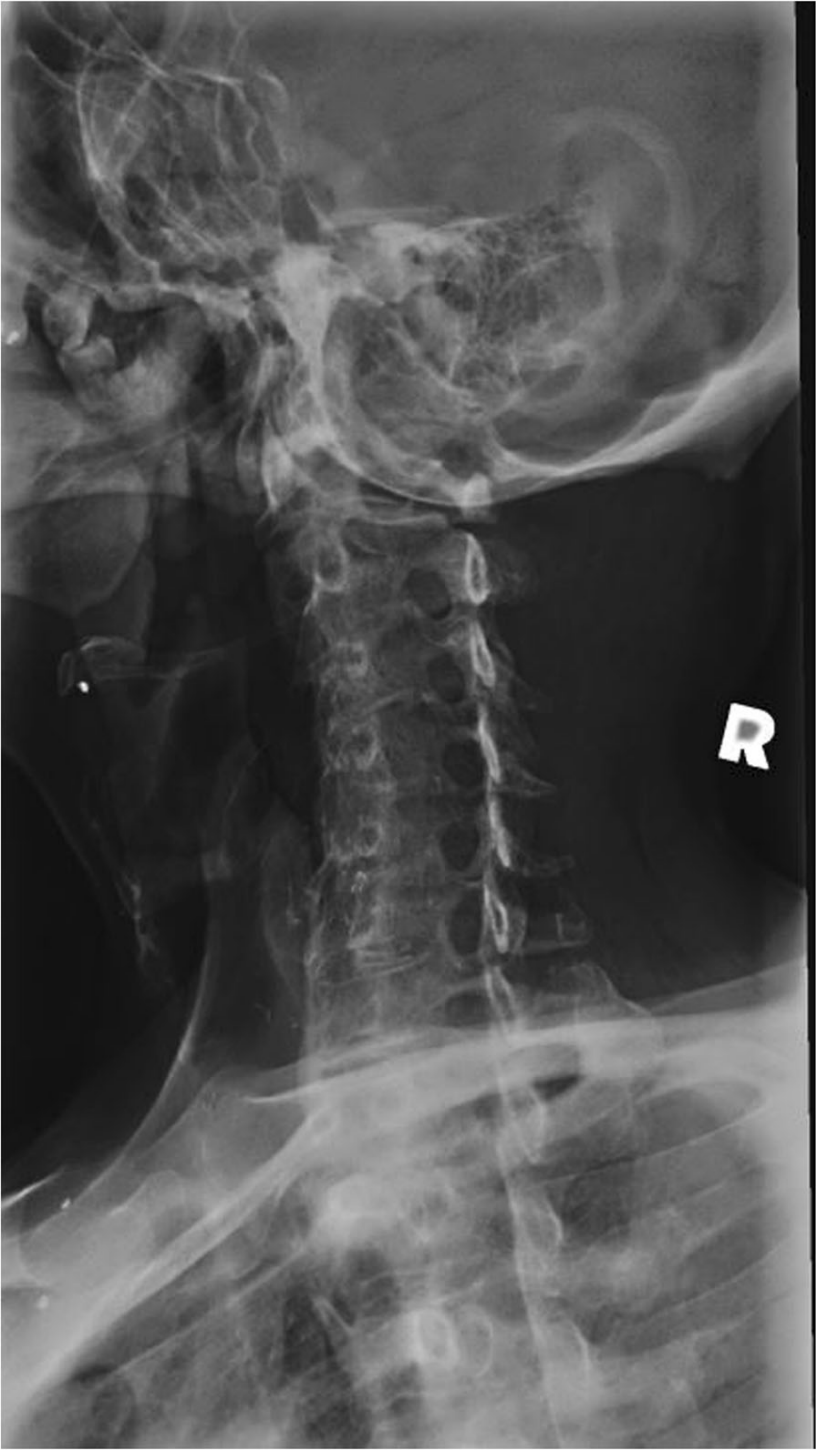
Right Oblique Plain Films, patent intervertebral foramina visualized
